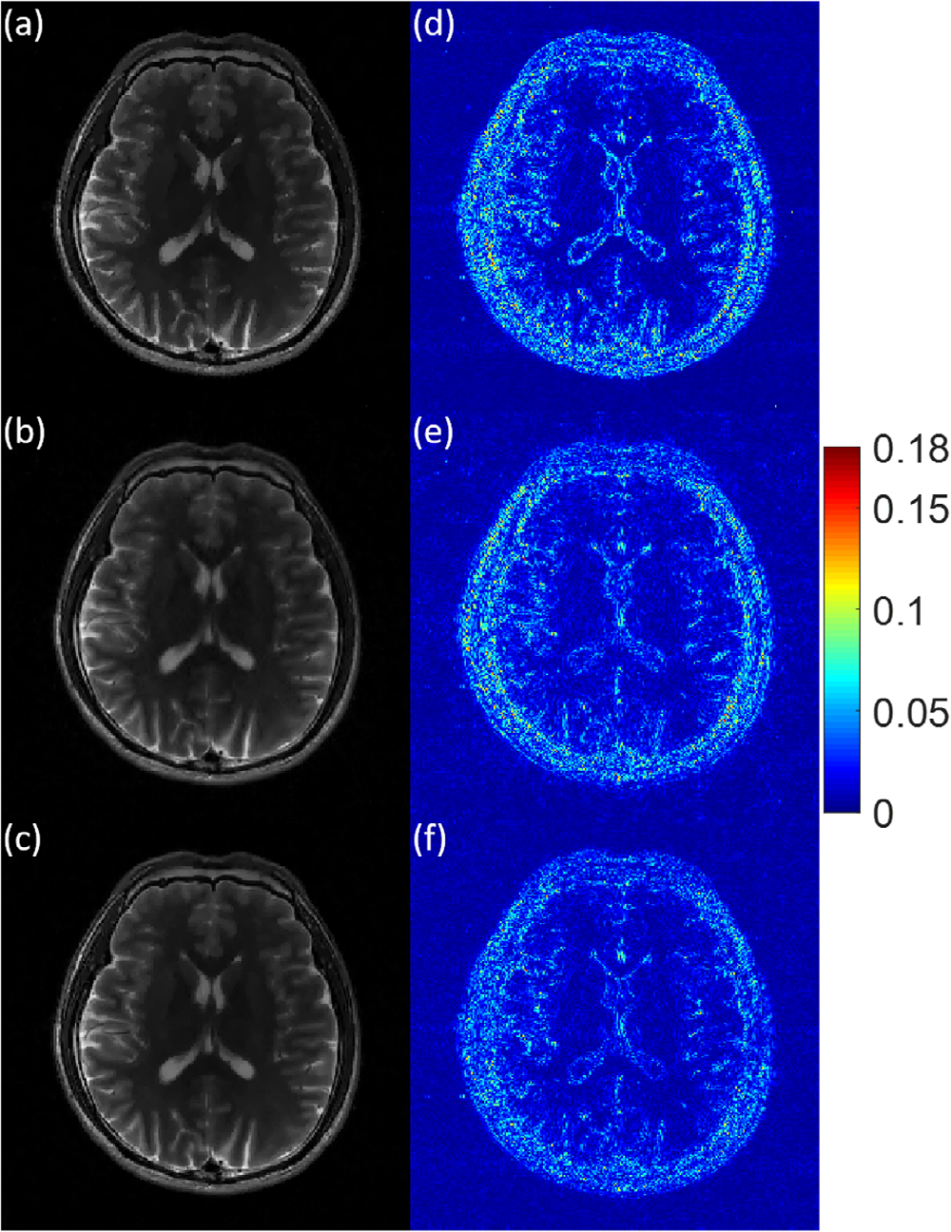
Reconstruction results of the 27th slice of the brain MRI 1. The parts of the figure (a)–(c) are the reconstruction results of MCTV‐L_2_, pFISTA, and TV+TFL_0_. The parts (d)–(f) are corresponding error images of (a)–(c).
